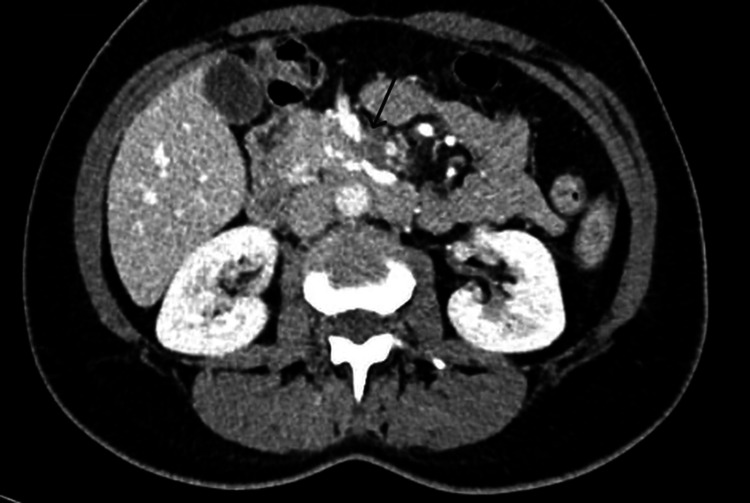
Contrast-enhanced CT scan from November 2024 showcasing an area of infiltrative densification (indicated by the arrow) with a mass-like appearance along the root of the mesentery, involving the adjacent vascular structures, measuring approximately 5 × 2.5 cm. It caused occlusion of a segment of the SMV, with visualization of some ectatic venous structures draining into the proximal segment of the vein, predominantly in the right abdominal quadrants. CT: computed tomography, SMV: superior mesenteric vein
